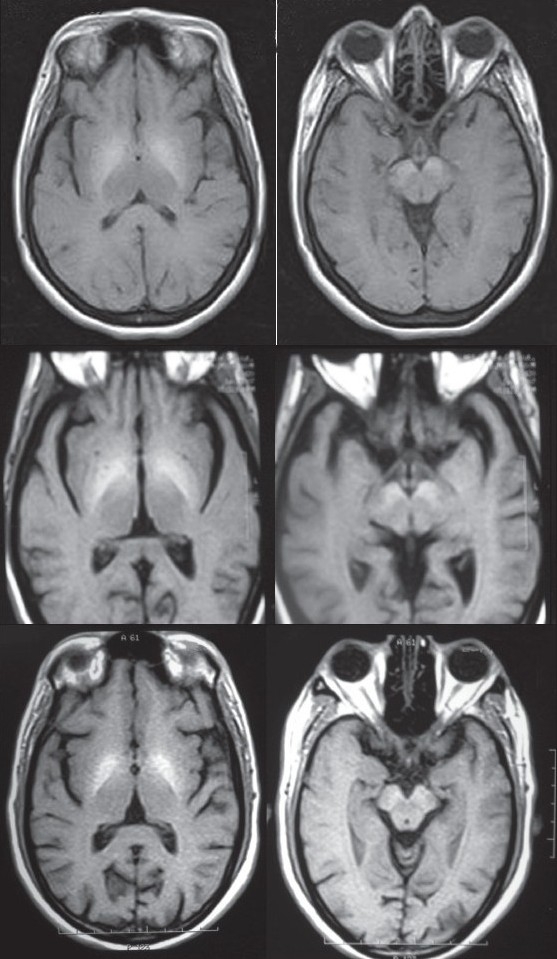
MRI Brain showing symmetrical hyperintensities in pallida and anterior midbrain in case 1 (A), case 2 (B) and case 3 (C).
